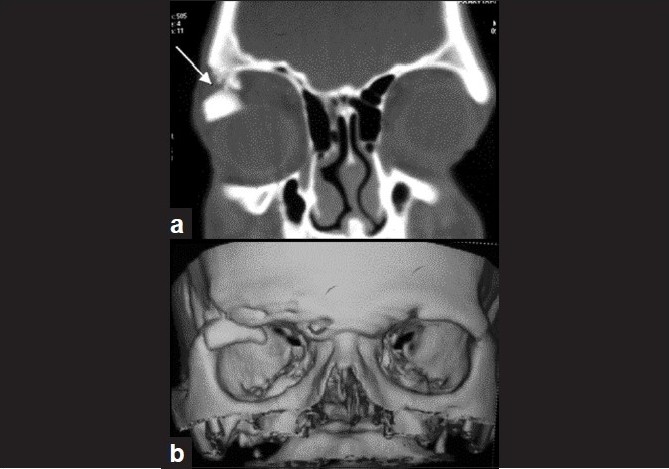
(a) CT scan (coronal view): RE roof fracture with inferior and medial displacement of fracture fragment (arrow) causing indentation of the right globe. (b) 3D reconstruction
